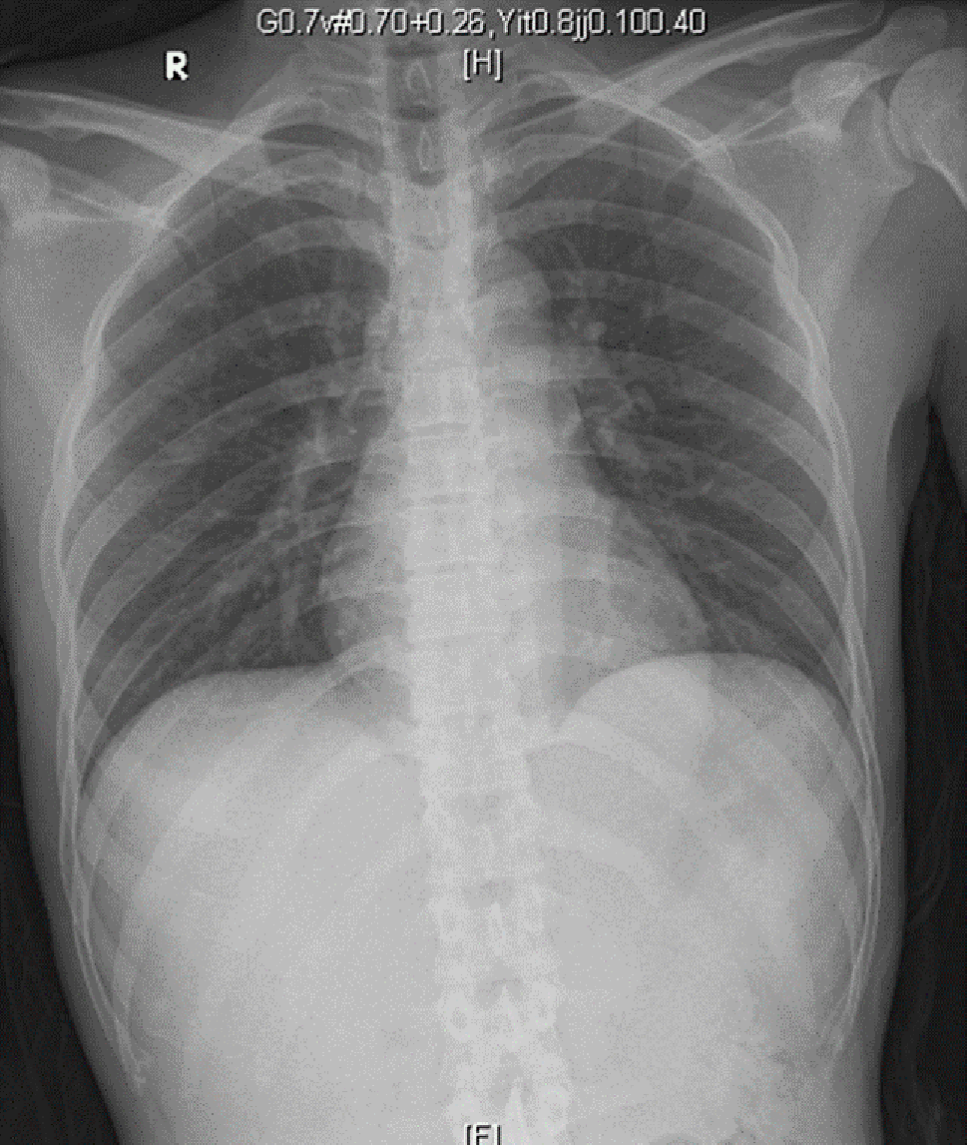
Chest X-ray showing mild increased lung markings in both lower lung fields
